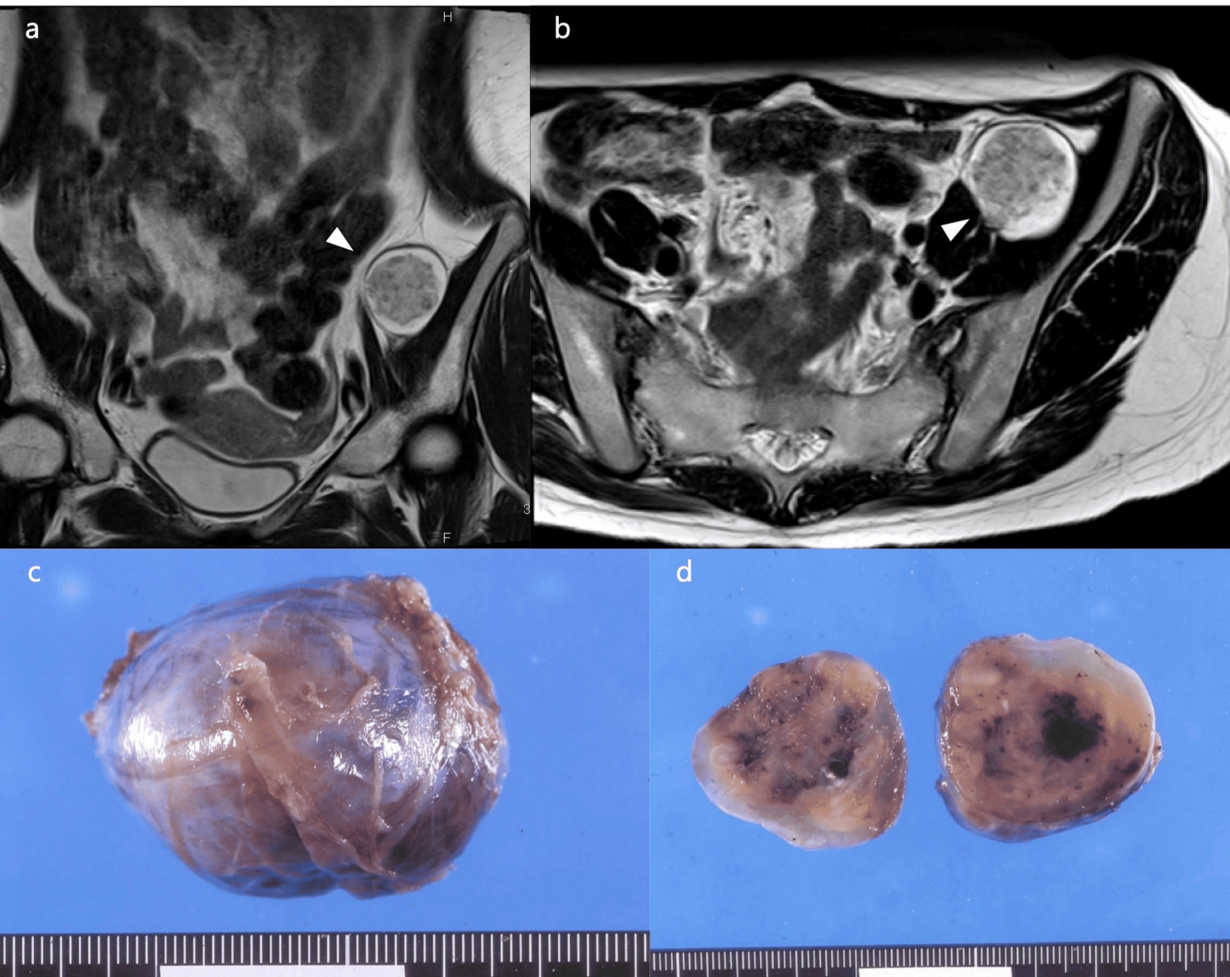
MRI images and the excised tumor (a) T2-weighted coronal image, white arrowheads indicate tumor; (b) T2-weighted axial image, white arrowheads indicate tumor; (c) Excised tumor; (d) Cross-section of excised tumor divided into two sections.
